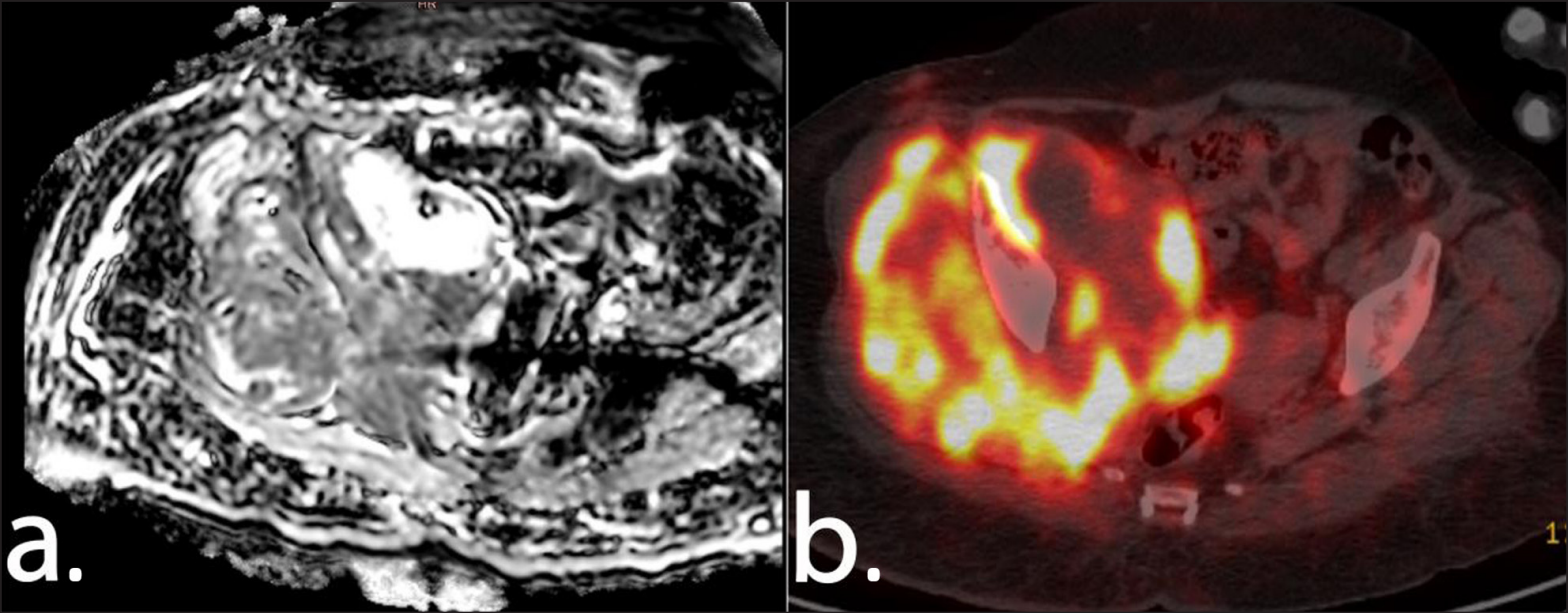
DWI (a) and FDG-PET/CT (b) images of a 51-year-old female with a soft tissue mass in the right iliac fossa. ADC_mean_, ADC_min_ and SUV_max_ of the lesion were 0.97 × 10^–3^mm^2^/s, 0.60 × 10^–3^mm^2^/s and 13.61, respectively. The lesion was diagnosed as high grade leiomyosarcoma after core needle biopsy.
